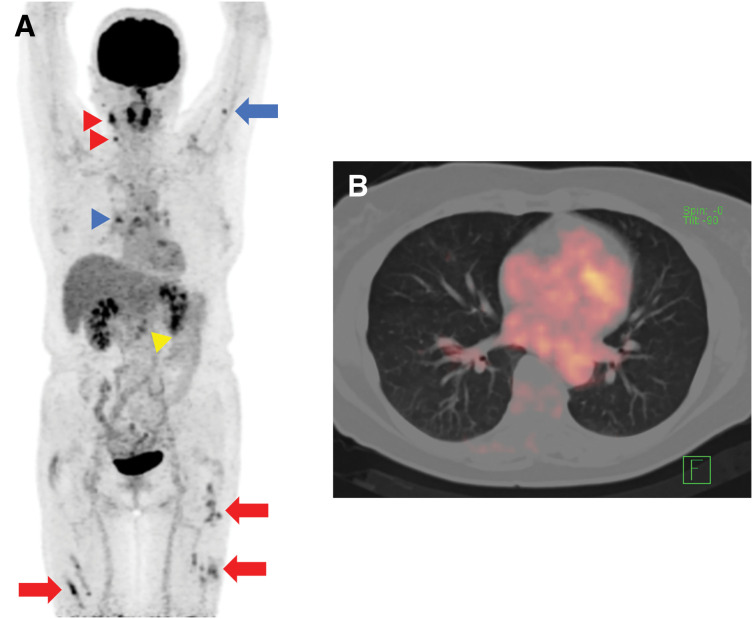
(A) On PET-CT, there were FDG accumulations at the cervical lymph nodes (red arrowheads), left upper arm (blue arrow), mediastinum and hilus (blue arrowhead), para-aortic lymph nodes (yellow arrowhead), and bilateral femoral muscles (red arrows). (B) There was no FDG uptake in the lung parenchyma. FDG, fluorodeoxyglucose
